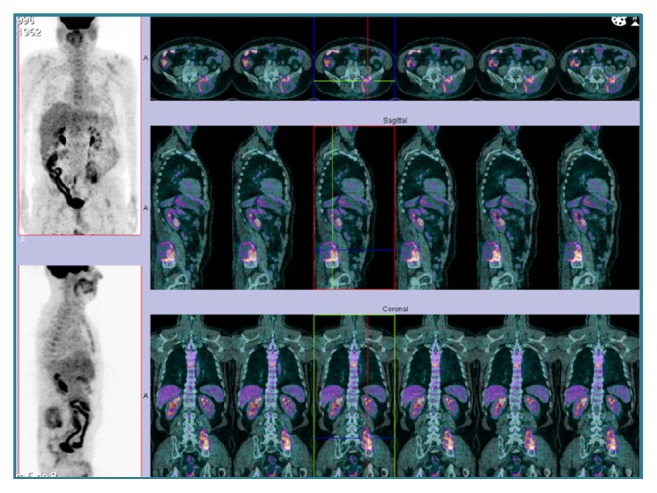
18F-FDG-PET revealing the pelvic lesion found on CT, without no other metabolically active lesions [Case 2].
